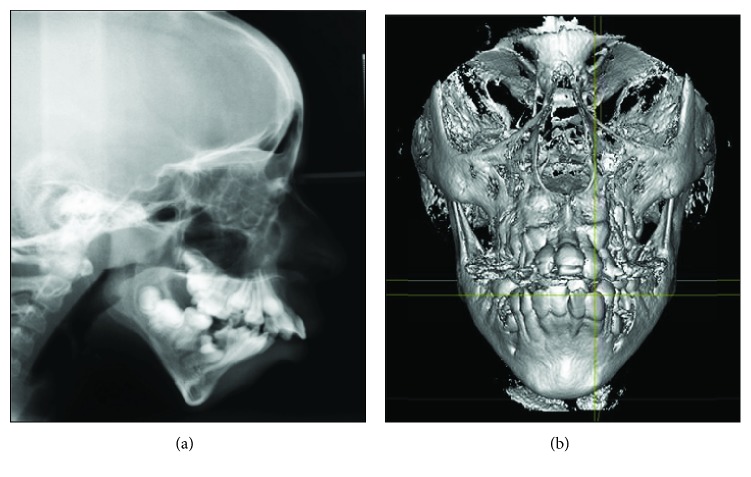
Images shows (a) lateral cephalogram and (b) cone beam computed tomography (CBCT) frontal view showing SMMCI.
